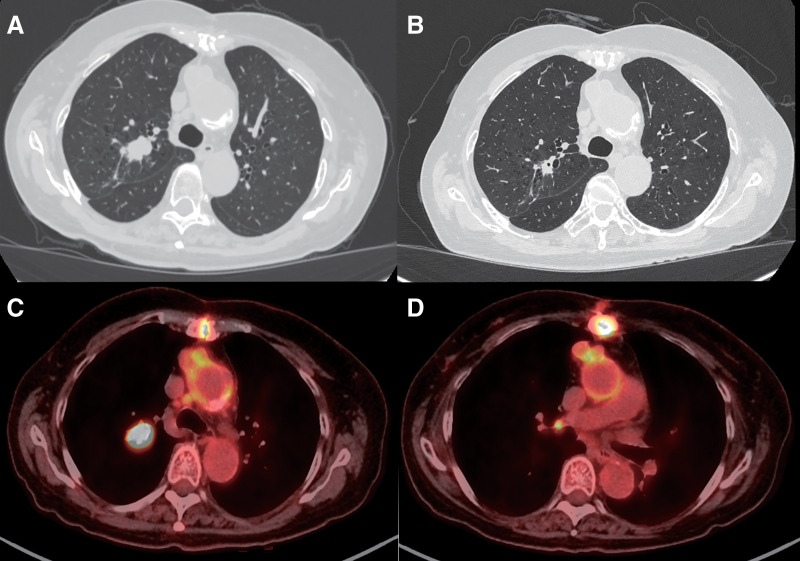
(A, B) Chest CT images obtained before (A) and after (B) neoadjuvant chemotherapy. A tumor in the right upper lobe decreased in size from 23 mm to 8 mm. (C, D) ^18^F-fluorodexyglycose positron emission tomography/computed tomography (^18^F-FDG PET/CT) findings before treatment. Increased FDG uptake is observed in the right upper lobe tumor (C; SUVmax = 29.43) and in the right hilar lymph node (D).
